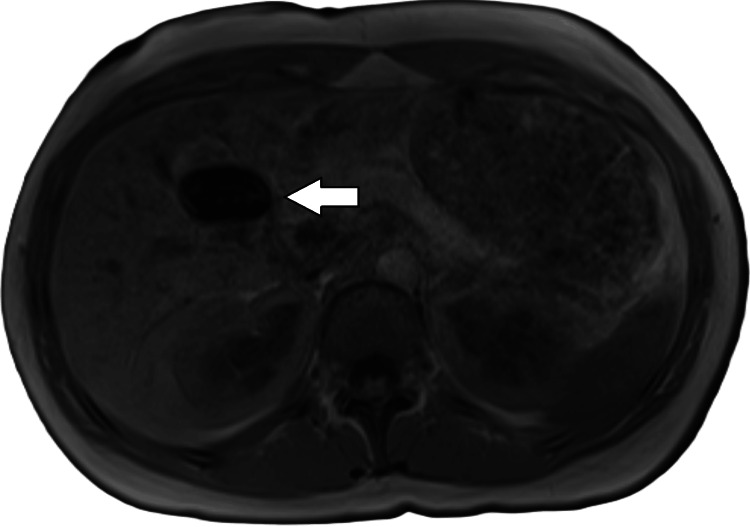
MRI abdomen without contrast MRI abdomen without contrast (MRCP) shows that the gallbladder is present and not distended (arrow).
